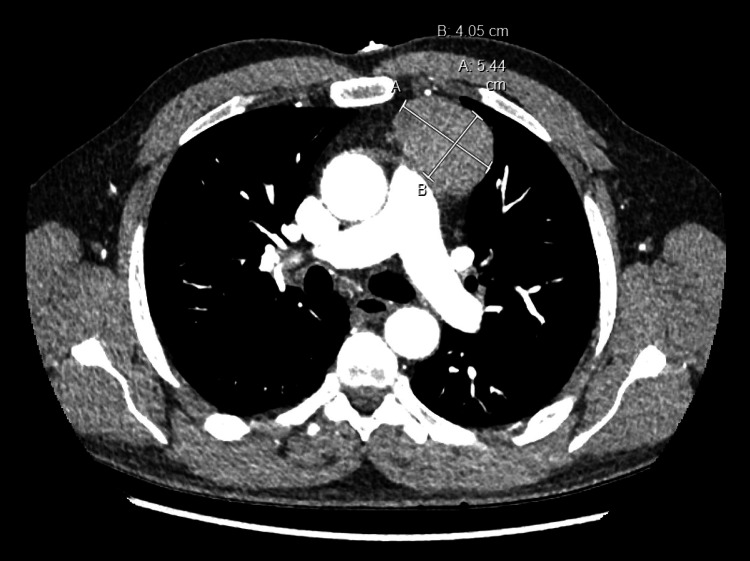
CT chest, abdomen, and pelvis angiography at initial presentation. A homogeneous soft tissue density anterior mediastinal mass, measuring 5.44 cm x 4.05 cm x 4.2 cm (not shown in the figure) is demonstrated on the axial slice.
